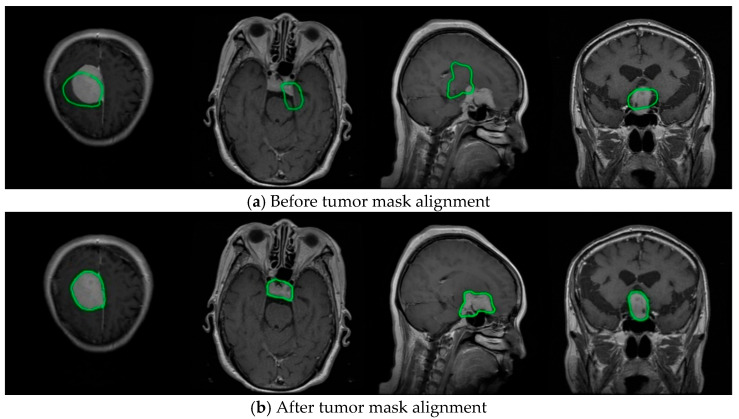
Dataset processing. (a) Original tumor masks (green contours) generated by YOLO before alignment; (b) Aligned tumor masks after registration. The green contours highlight the spatial adjustment of labels across MRI slices.
